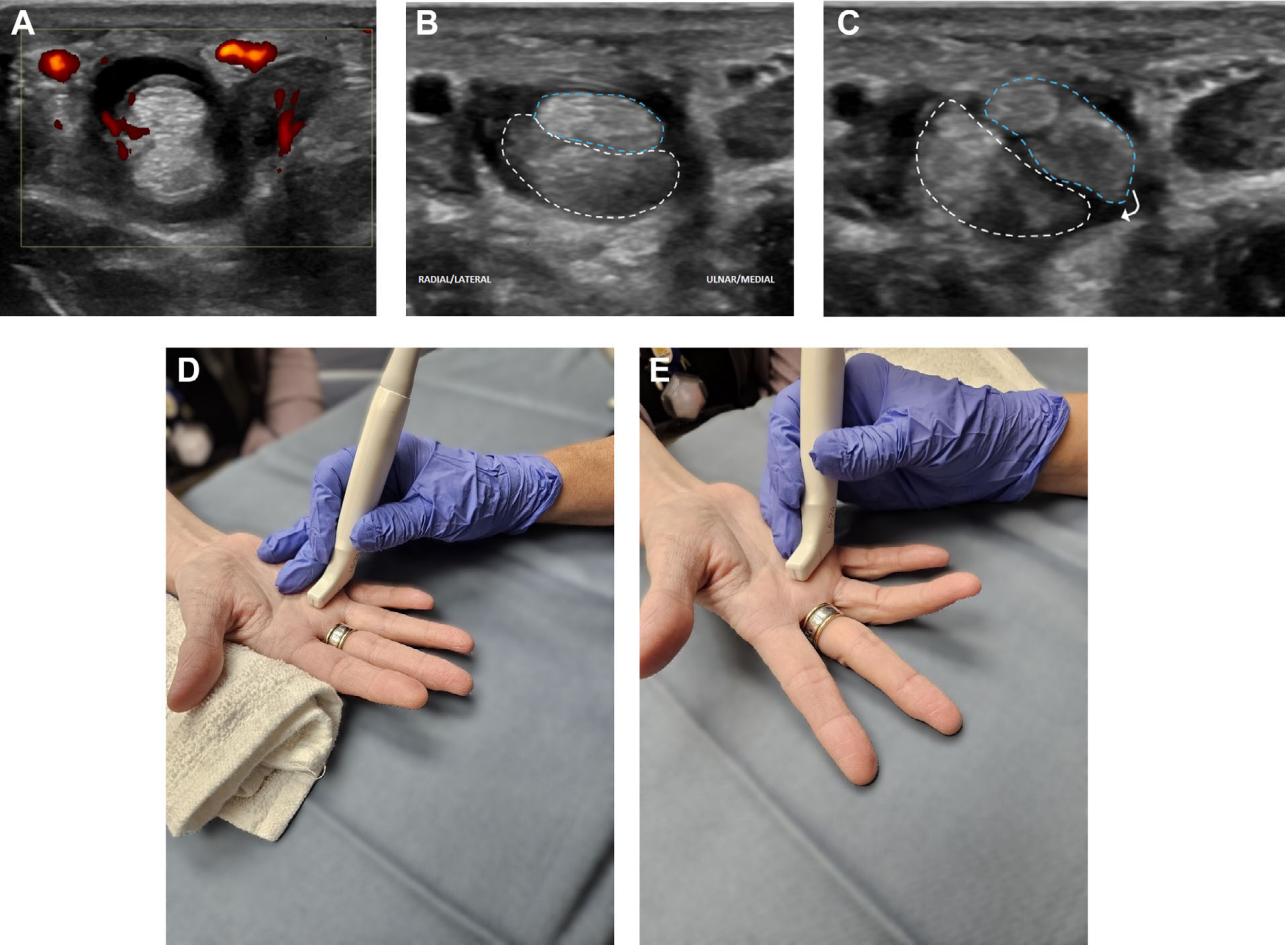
Active flexor tenosynovitis with intrasheath subluxation of the FDS and FDP tendons in a 48‐year‐old woman. A–C, Short‐axis images of the fourth digit flexor tendons demonstrating active tenosynovitis with hyperemia on Doppler imaging (A). In B, there is a normal relationship of the FDS (blue outline) and FDP (white outline) tendons at rest. In C, intrasheath subluxation is noted during active dynamic maneuvers. C–E, Pictures showing the US technique with the transducer positioned in the short axis, at the level of the fourth metacarpophalangeal joint/A1 pulley, at rest in D and with active dynamic flexion in E.
